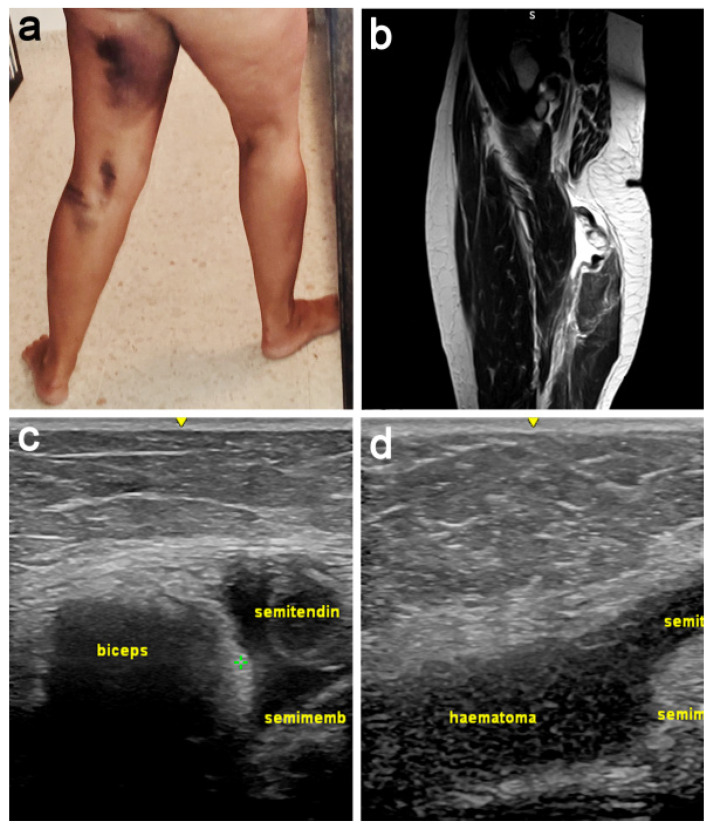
Preoperative description of the case. (a) Image of the haematoma two days after the accident, accompanied by lancinating pain with paresthesia similar to radicular pain. (b) MRI confirming the injury; the conjoint tendon was retracted 7 cm and the semimembranosus tendon 10 cm, with a significant accumulation of free fluid. (c,d) Ultrasound also showed complete disinsertion of both tendons, as well as their retraction.
